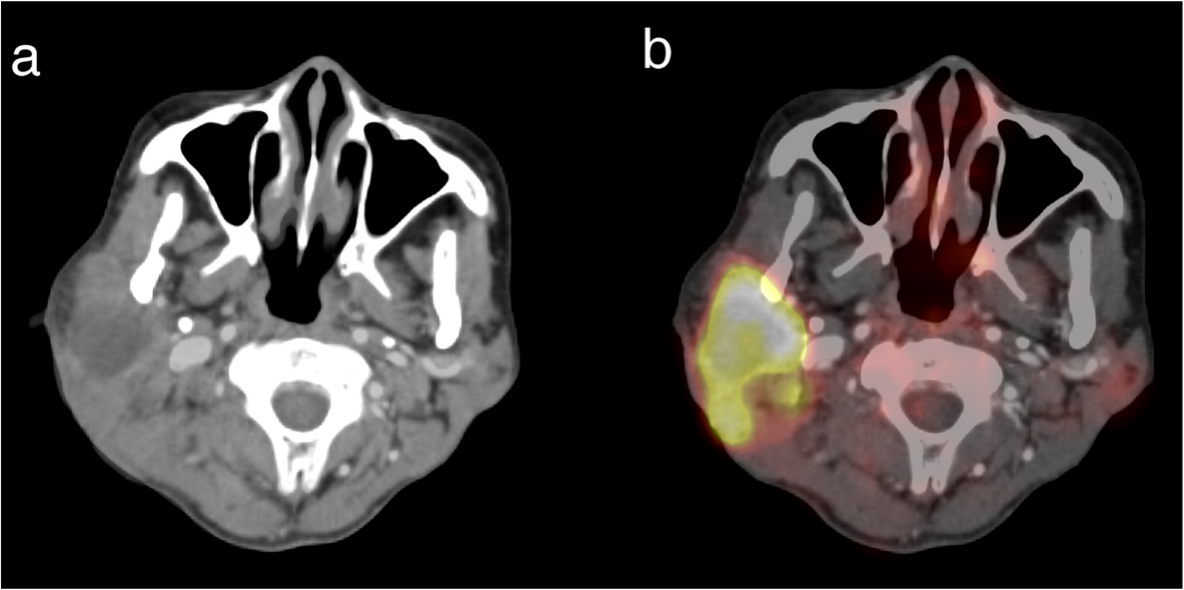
The CT of PET/CT and CECT datasets were registered precisely (a). The SUVmax threshold was adjusted based on the registered CECT and the fused images between PET and CECT are shown (b)
